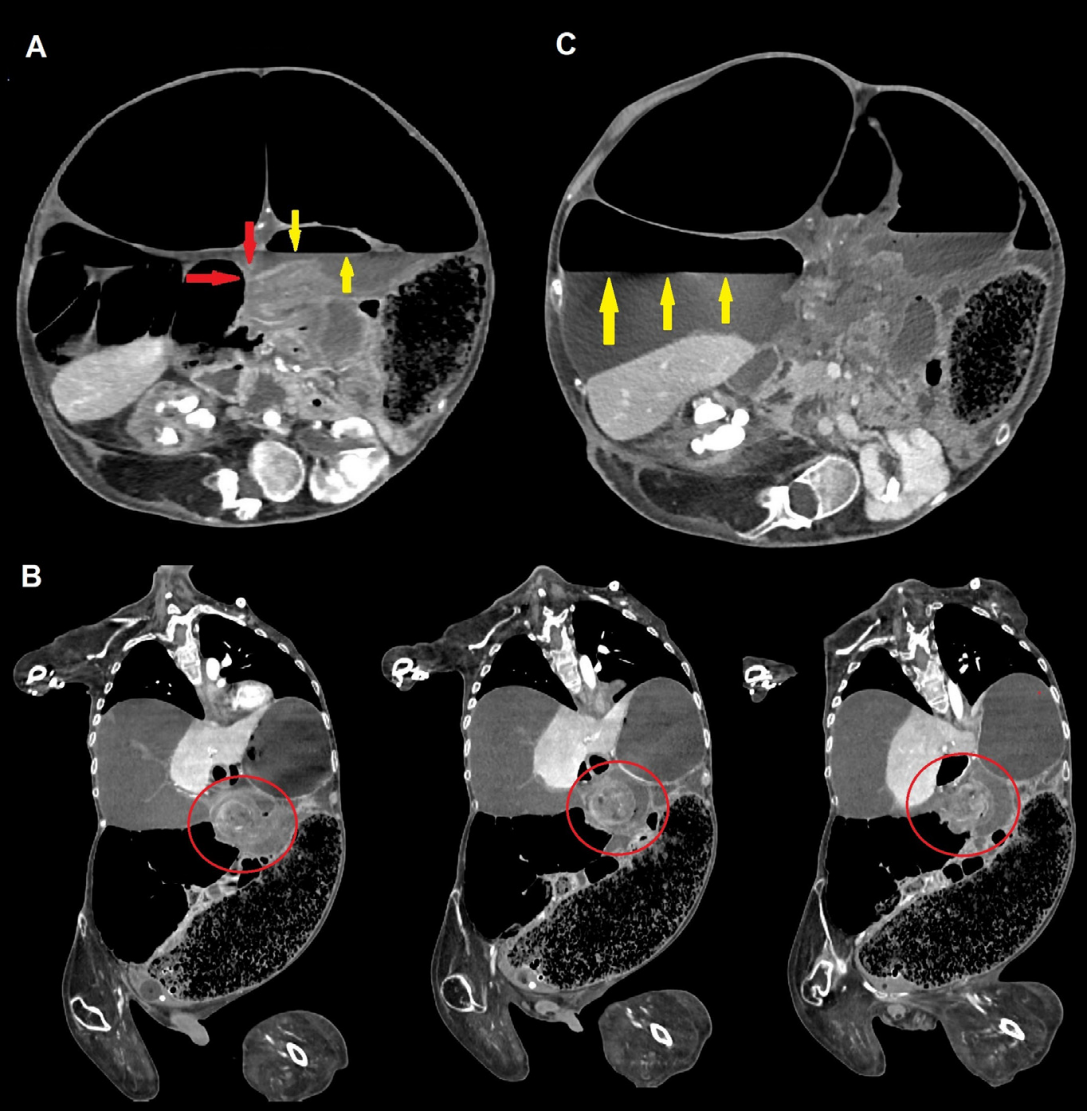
Contrast-enhanced CT scans of the abdomen demonstrating volvulus of the transverse colon. Axial scans (A) show an abrupt caliber change (“*beak sign*” [red arrows], common in many types of mechanical ileum) representing the site of occlusion, associated with proximal air-fluid level (yellow arrows). Coronal view (B), obtained with multiplanar reconstructions (MPR), demonstrates the same loop twisting around the long axis of its meso (“*whirl sign*” [red circles]); most of the right colon is displaced, as well as jejunal loops (medially), due to partial involvement of the root of the mesentery. Significant right colic dilatation (with atony) is present, with a caliber of caecum measuring more than 17 cm and an evident air-fluid level (C [yellow arrows]).
